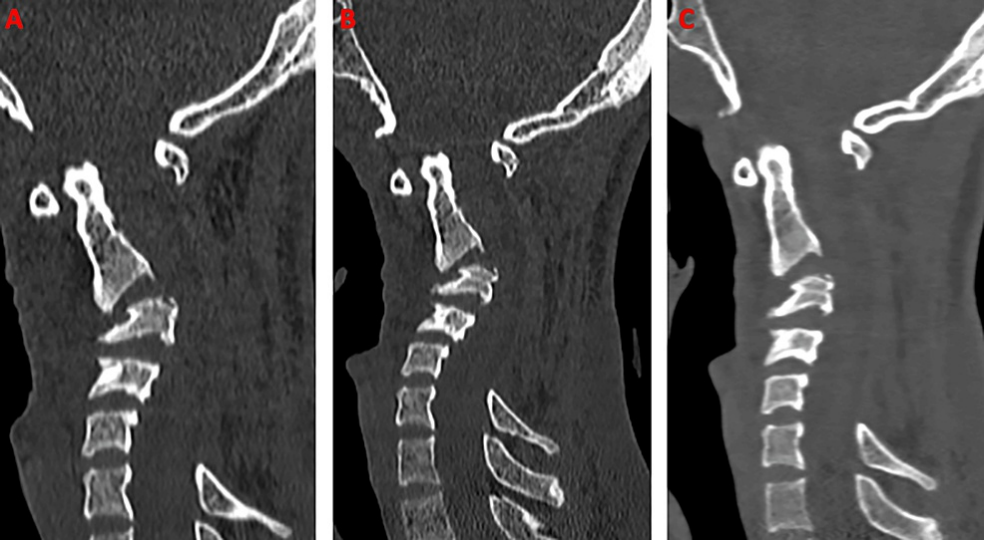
CT sagittal view showing progressive reduction of C3 retrolisthesis and cervical kyphosis with a gradual increment in the traction weights. (A) 3 kg. (B) 5 kg. (C) 8 kg.
